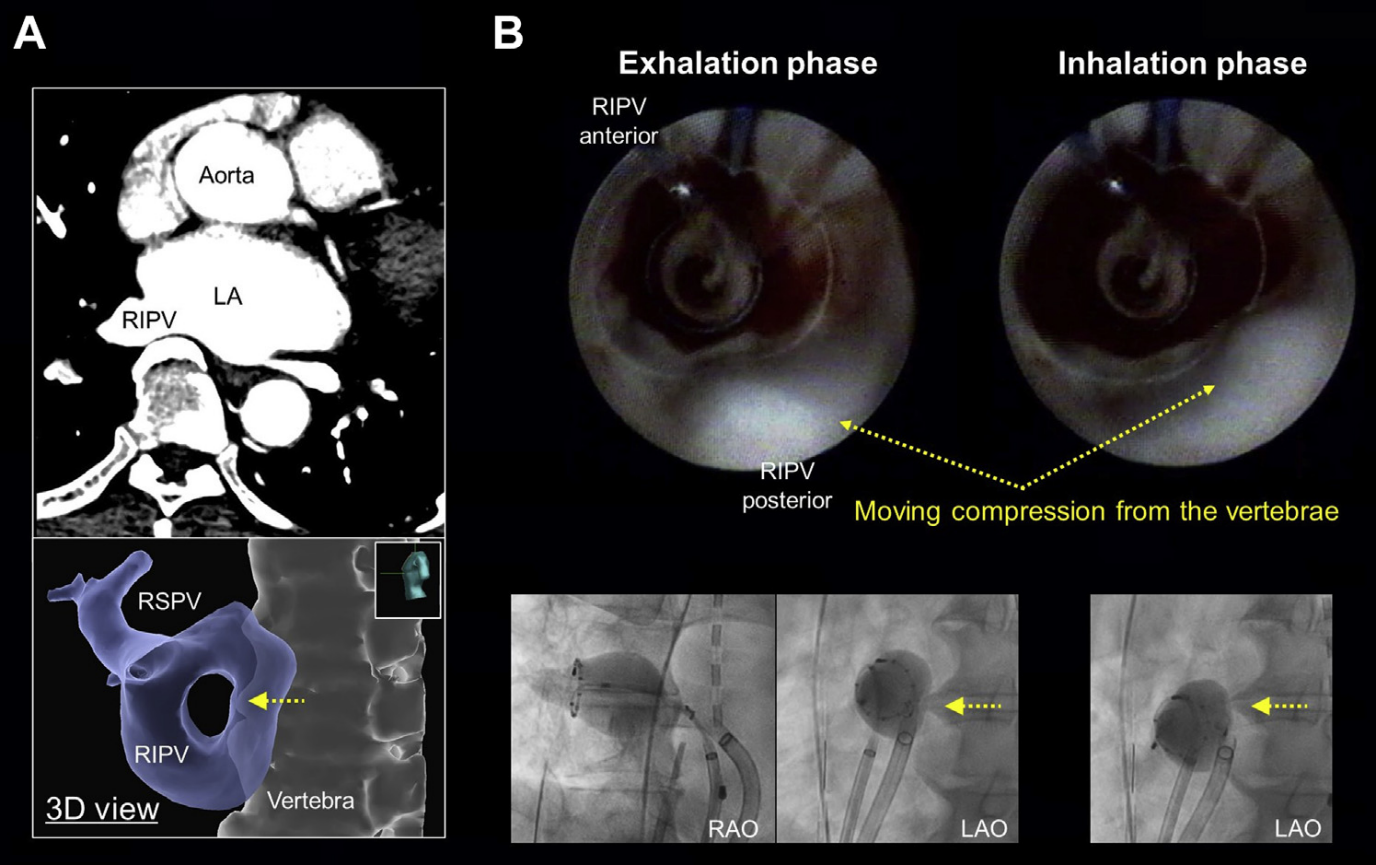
A: Enhanced computed tomography demonstrating strong contact from the vertebra (top panel) and 3-D view of the vertebra compressing the right inferior pulmonary vein (RIPV) antrum. B: Endoscopic view of the RIPV antrum is shown in top panel. The compression area from the vertebra moves corresponding to the respiratory movements. The bottom panel shows the fluoroscopic image of the laser balloon and vertebra during respiratory movements. The compression on the posterior region of the RIPV antrum was stronger on the inferior side (exhalation phase) than superior side (inhalation phase). LA = left atrium; LAO = left anterior oblique; RAO = right anterior oblique; RSPV = right superior pulmonary vein.
